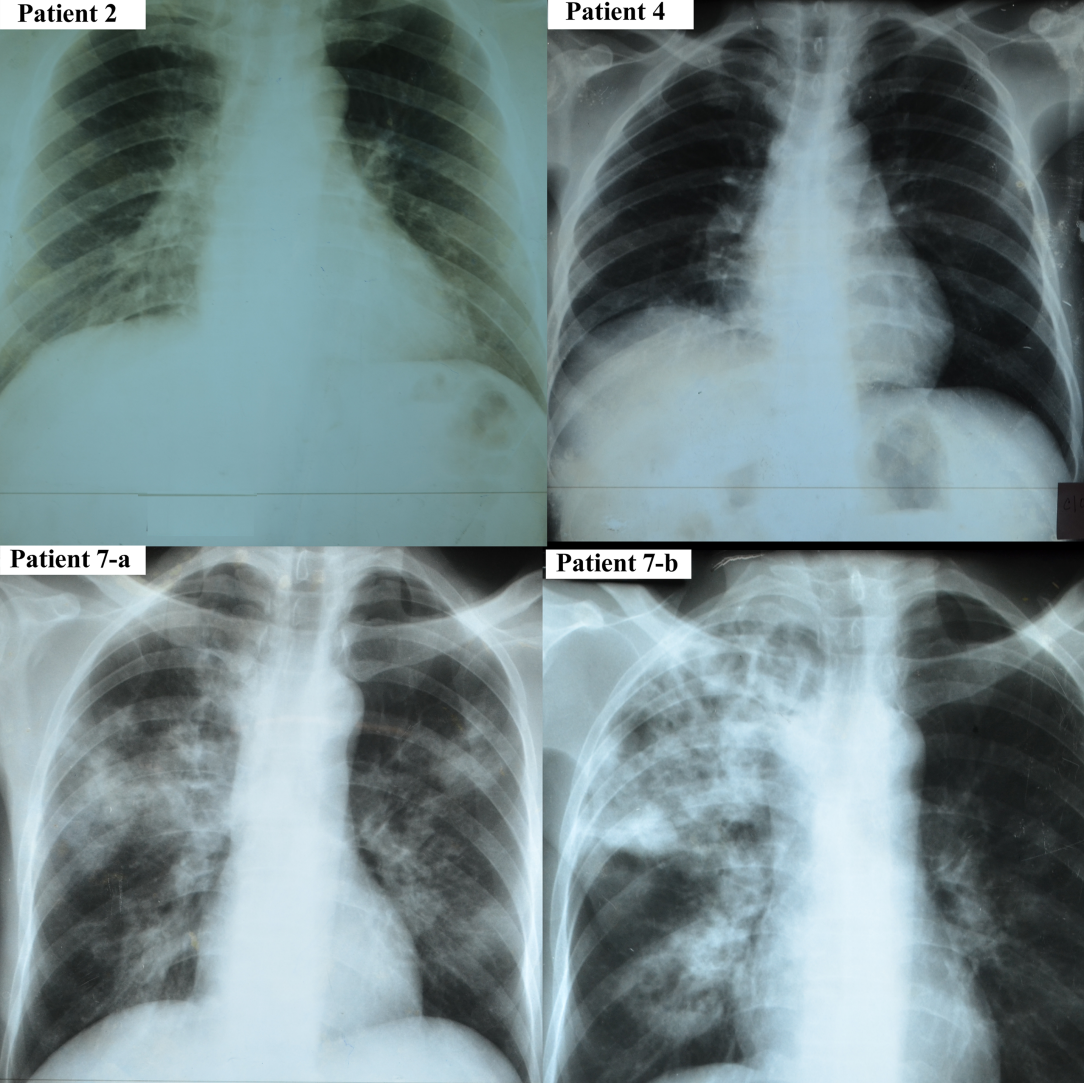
Chest radiography. Plain films taken on hospital day 1 (patient 2, 4, 7a) and hospital day 9 (patient 7-b).
